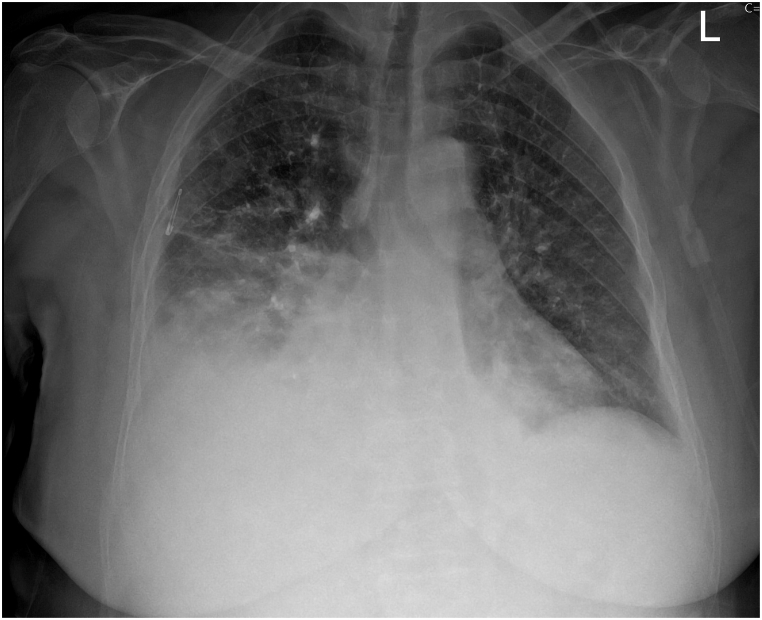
Chest radiograph on postoperative day 5 showing increased right-sided opacities and pleural effusion, with new infiltrates consistent with evolving lung pathology.
